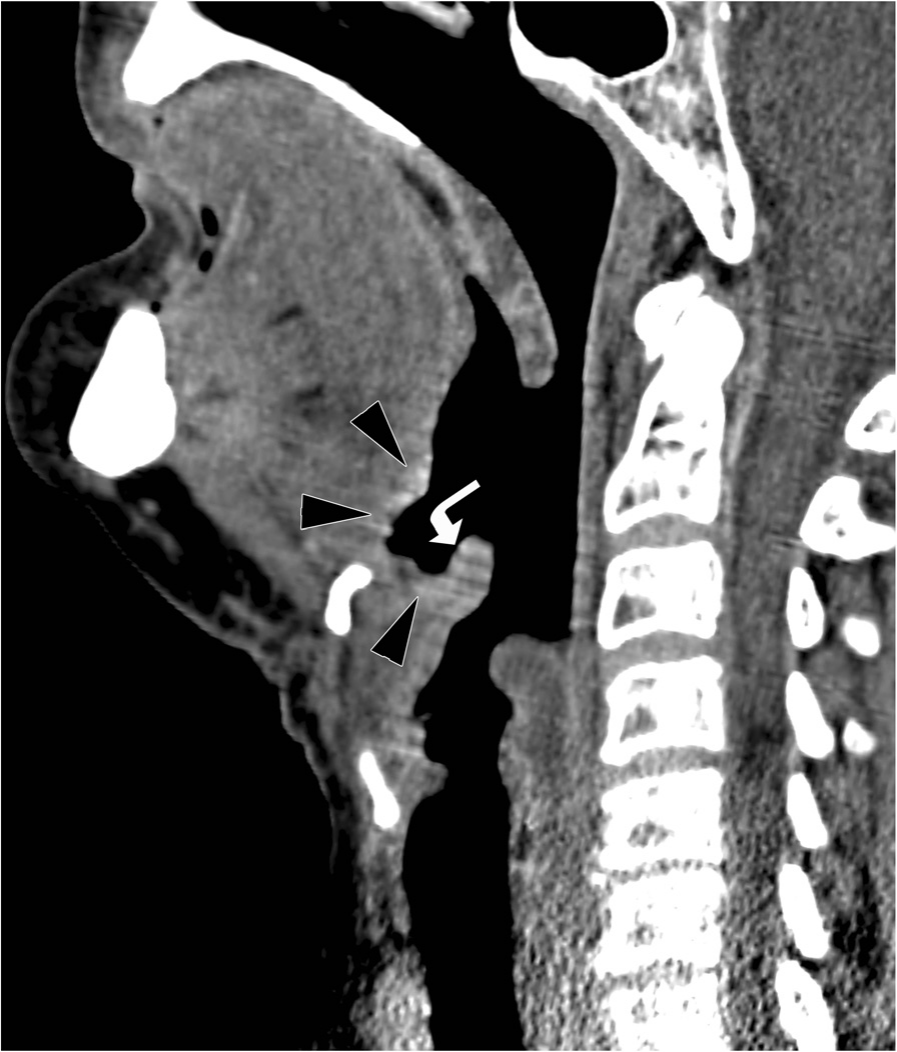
Patient 2. Pretreatment sagittal contrast-enhanced CT-image shows ulceration with marginal enhancement in the BOT midline (*arrowheads*); the soft tissue thickening extends on the lingual surface of the epiglottis (*curved arrow*)
